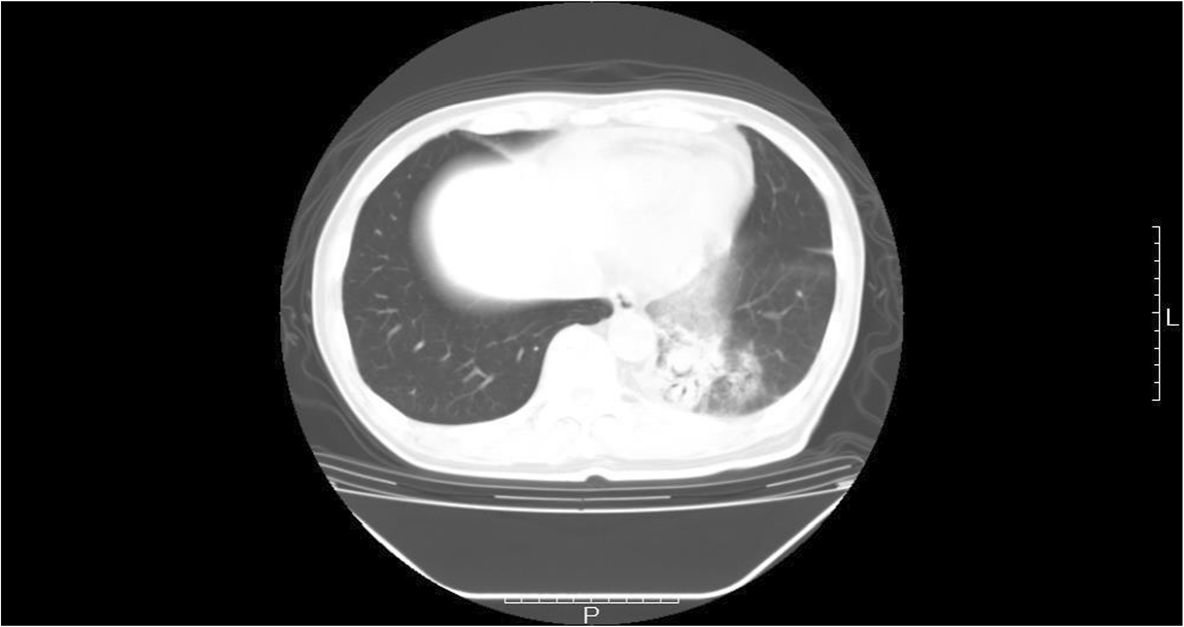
Chest computed tomography on 21 January 2009. The mass at S10 of the left lung is not detected
